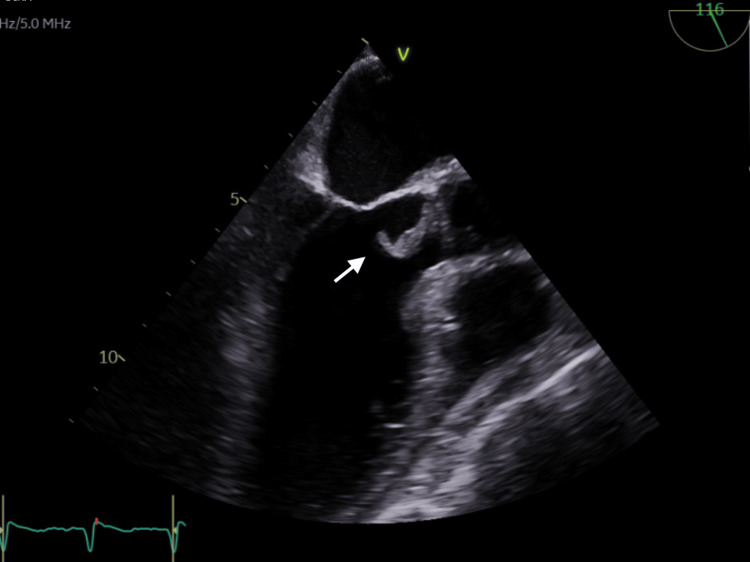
Transesophageal echocardiogram; mid-oesophageal long axis view in systole showing a 0.63 cm x 1.59 cm vegetation (white arrow) attached to the non-coronary cusp.
